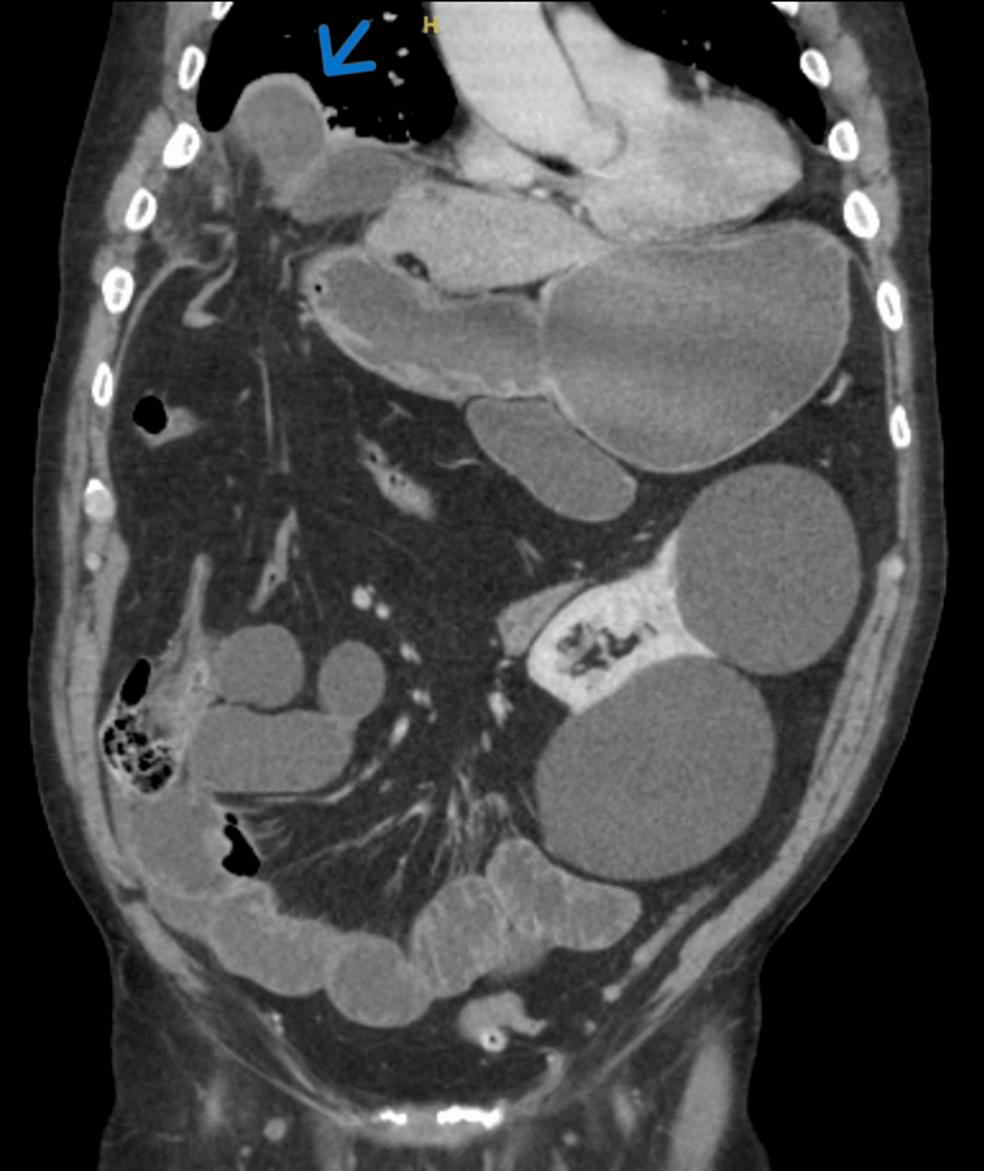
Abdominal and pelvic CT scan demonstrating small bowel obstruction and right-sided diaphragmatic hernia (blue arrow) CT: computed tomography
